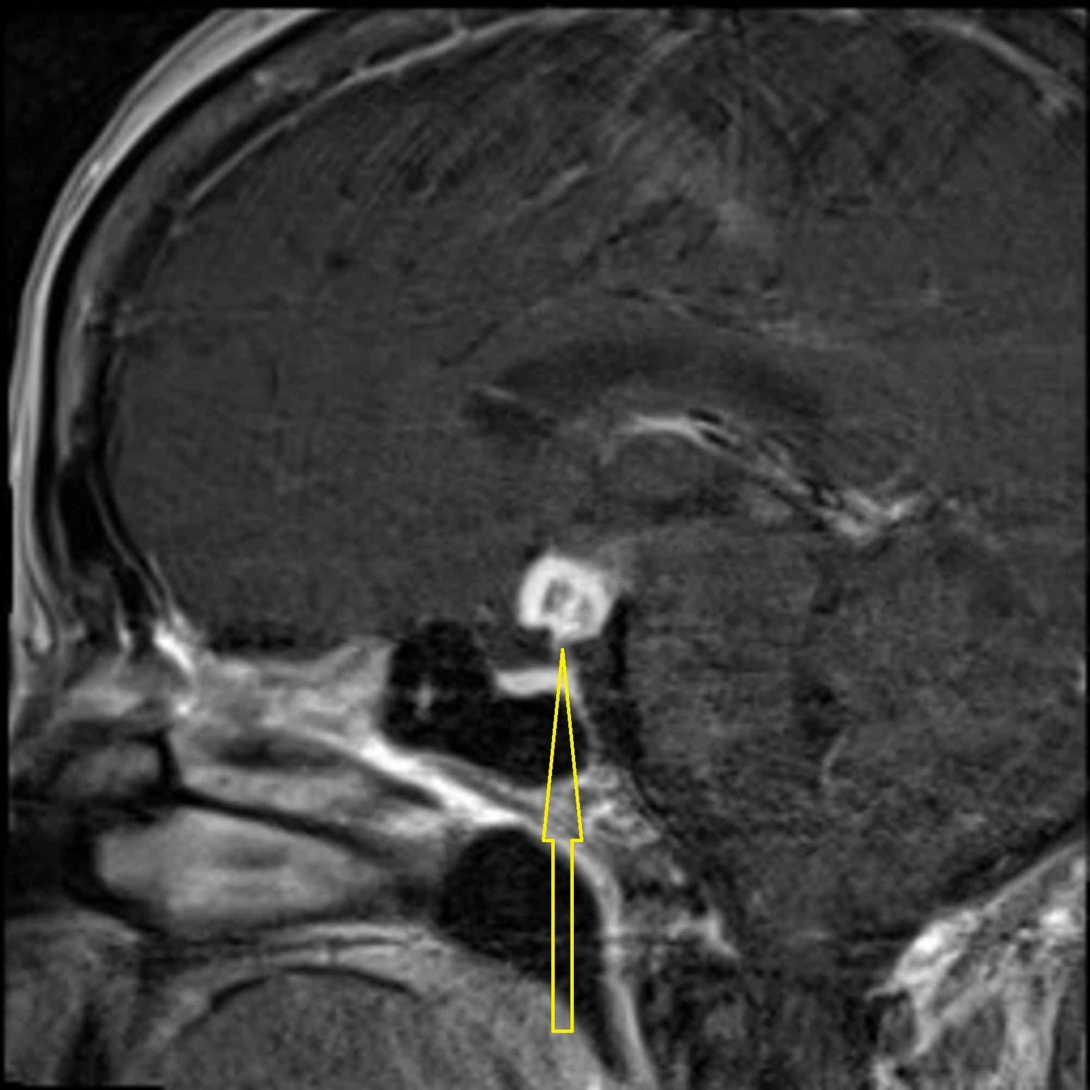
MRI of the pituitary w/wo contrast showed suprasellar mass arising from either the hypothalamus or less likely the chiasm.
